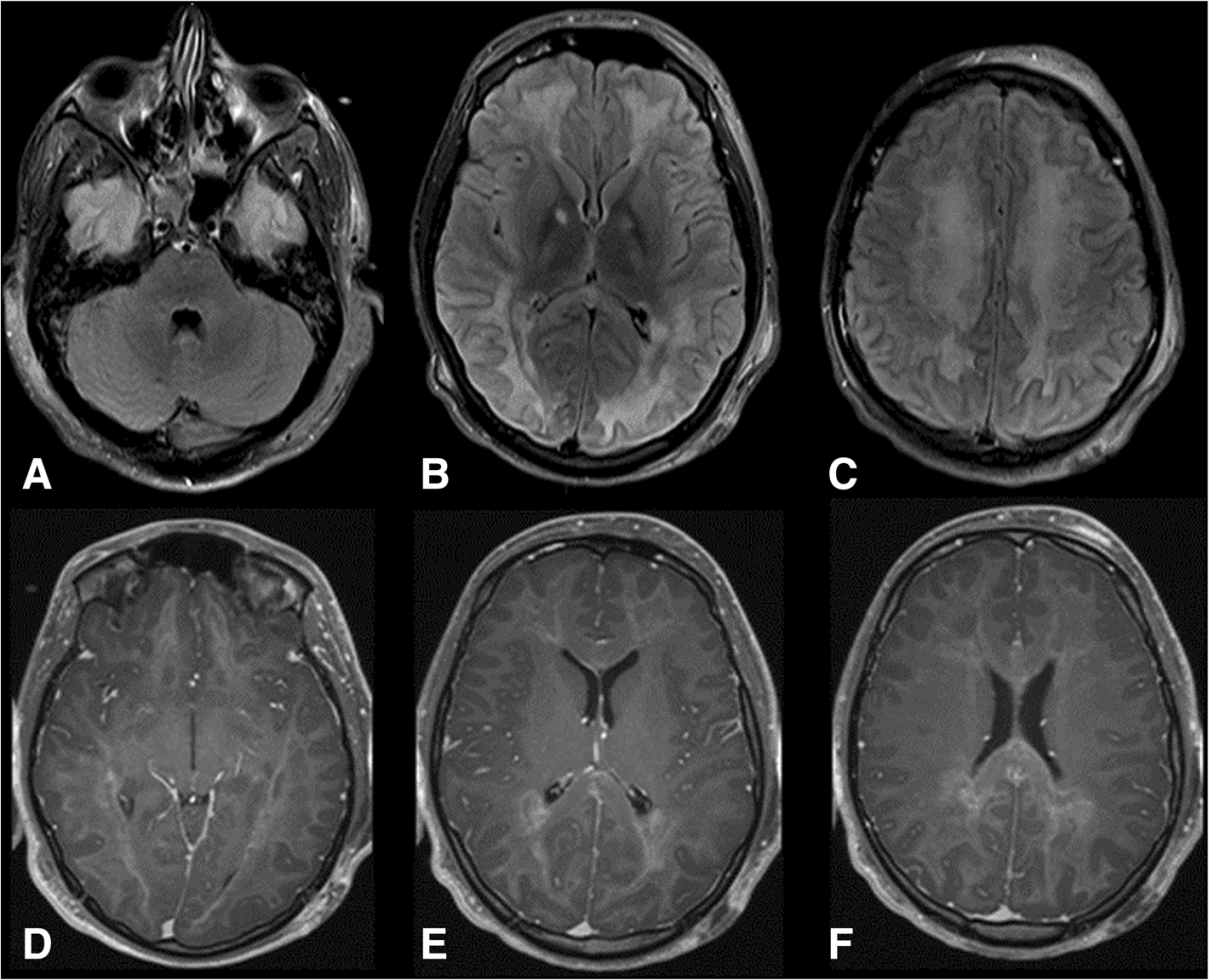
Magnetic resonant images of the brain. A-C) FLAIR images shows diffusely increased signal intensity in cerebral white matter. Small hyperintense foci are seen in globus pallidus bilaterally which is likely ischemic secondary to hypoxia. Left frontal scalp hematoma is seen. D-F) Post contrast T1 weighted images show minimal patchy enhancement is seen in cerebral white matter.
